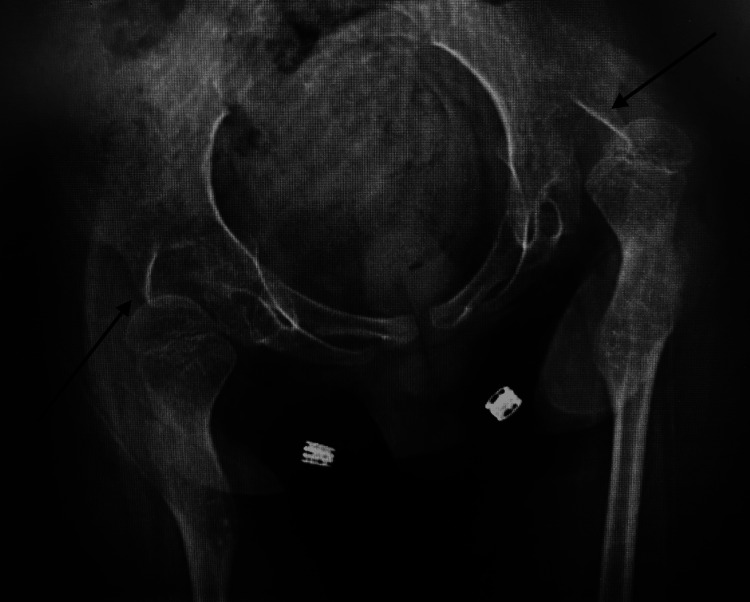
AP view pelvis radiograph with bilateral hip dislocation present as shown by the arrows AP: Anteroposterior
